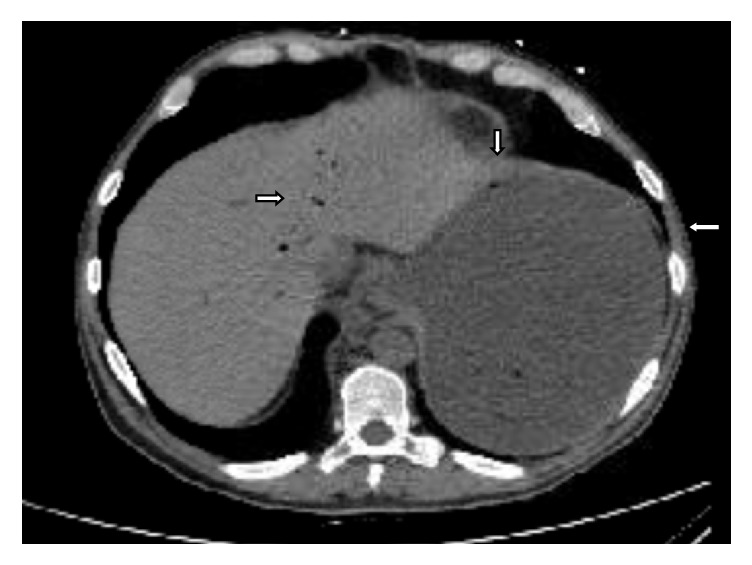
Abdominal CT scan showing stomach distension and limited gas within the wall of the stomach and in the portal venous system.
